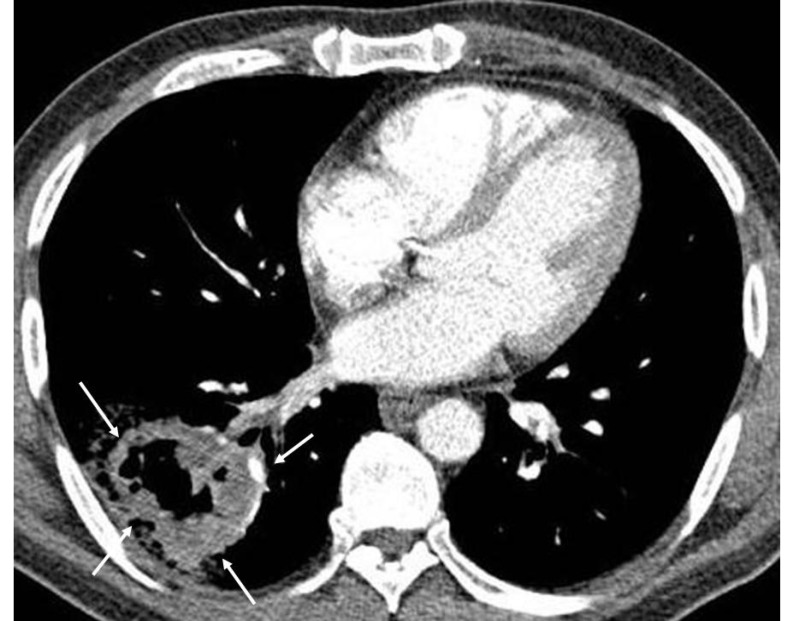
Contrast enhanced CT of a 59 years old male smoker with bronchogenic carcinoma shows a large mass (arrows) located peripherally in the lower lobe of the right lung. Mass has an irregular margin and contains air suggesting presence of necrotic changes. In addition to a malignancy, necrotic pulmonary lesion can also be encountered in the setting of pyogenic, mycobacterial and fungal infection, metastasis, Wegener’s granulomatosis, infarction etc
